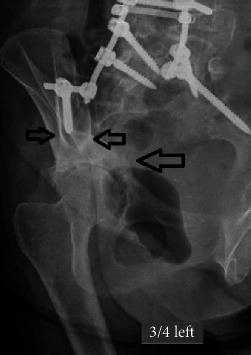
Oblique left Judet roentgenogram of the right hip 18 months postoperatively showing the transverse acetabular fracture. The left hemipelvis and particularly the acetabulum are intact. There is a radiolucent line around the tip of the left iliac screw. The right iliac screw has a central correct between the anterior and posterior iliac cortices.
